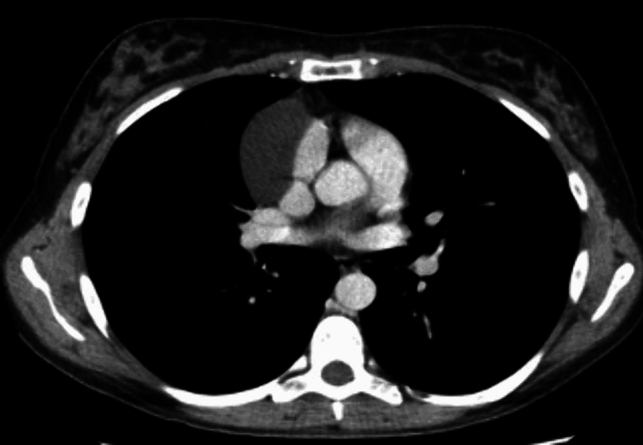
(Thoracic CT scan: thymic cyst)
